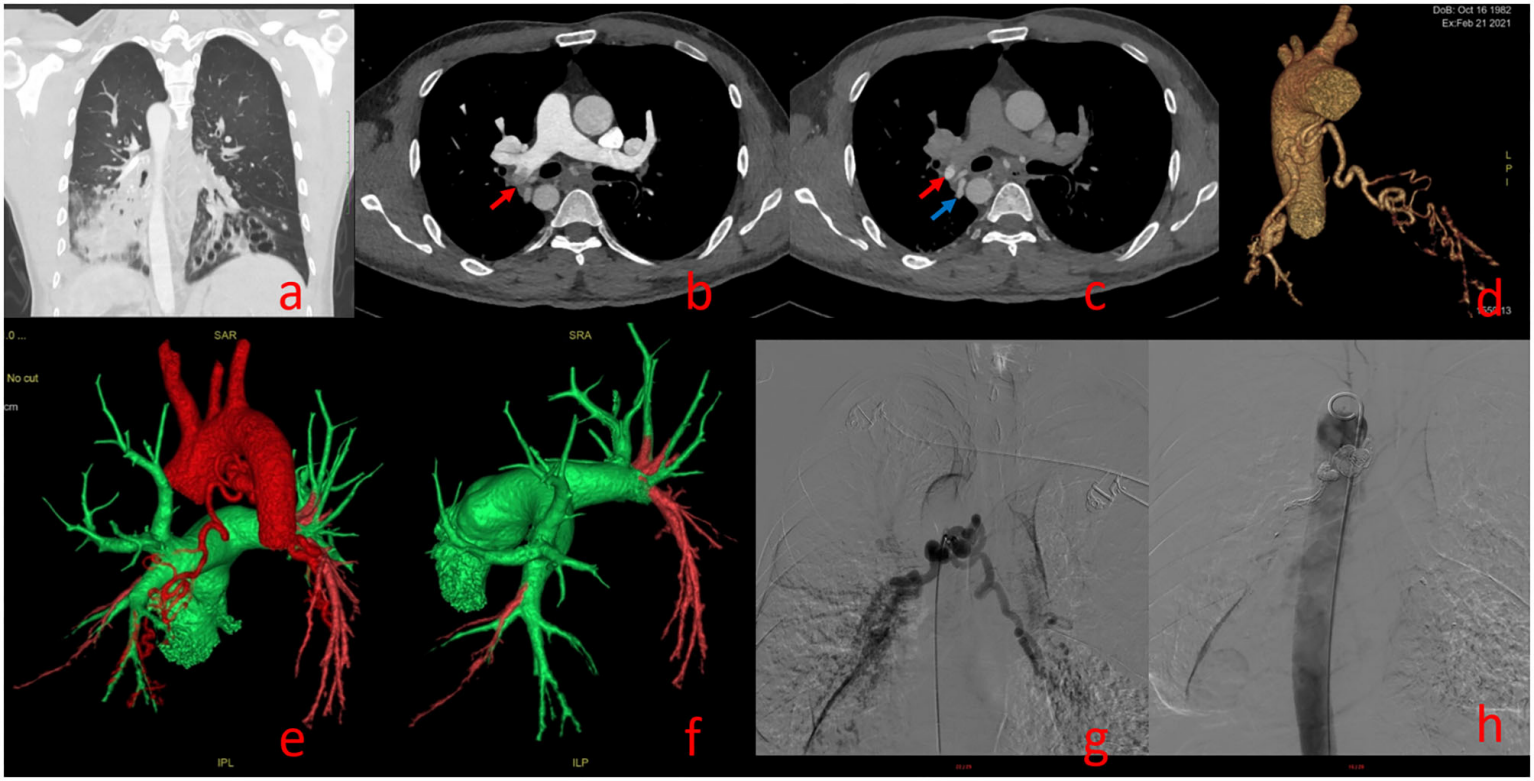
Systemic-Pulmonary Shunt artifacts (Supplementary Video 6). A 39-year-old man with Kartagener syndrome underwent dual phase-computed tomography pulmonary angiography. Bronchiectasis of lower lobes of both lungs in (a). (b) (pulmonary artery phase): Filling defect can be seen in the right lower pulmonary artery [red arrow in (b)]; (c) (aortic phase): the filling defect shown in (b) shows high density in (c) [red arrow in (c)], and its density is similar to that of the adjacent bronchial artery [blue arrow in (c)]; Three dimensional reconstruction showing thickening of bronchial artery trunk in (d); (e,f) show bronchial-pulmonary artery shunt, Green represents the normal blood flow of pulmonary artery and red represents the normal blood flow of aorta; bronchial-pulmonary artery shunt confirmed by angiography and treated by bronchial artery embolization in (g,h).
